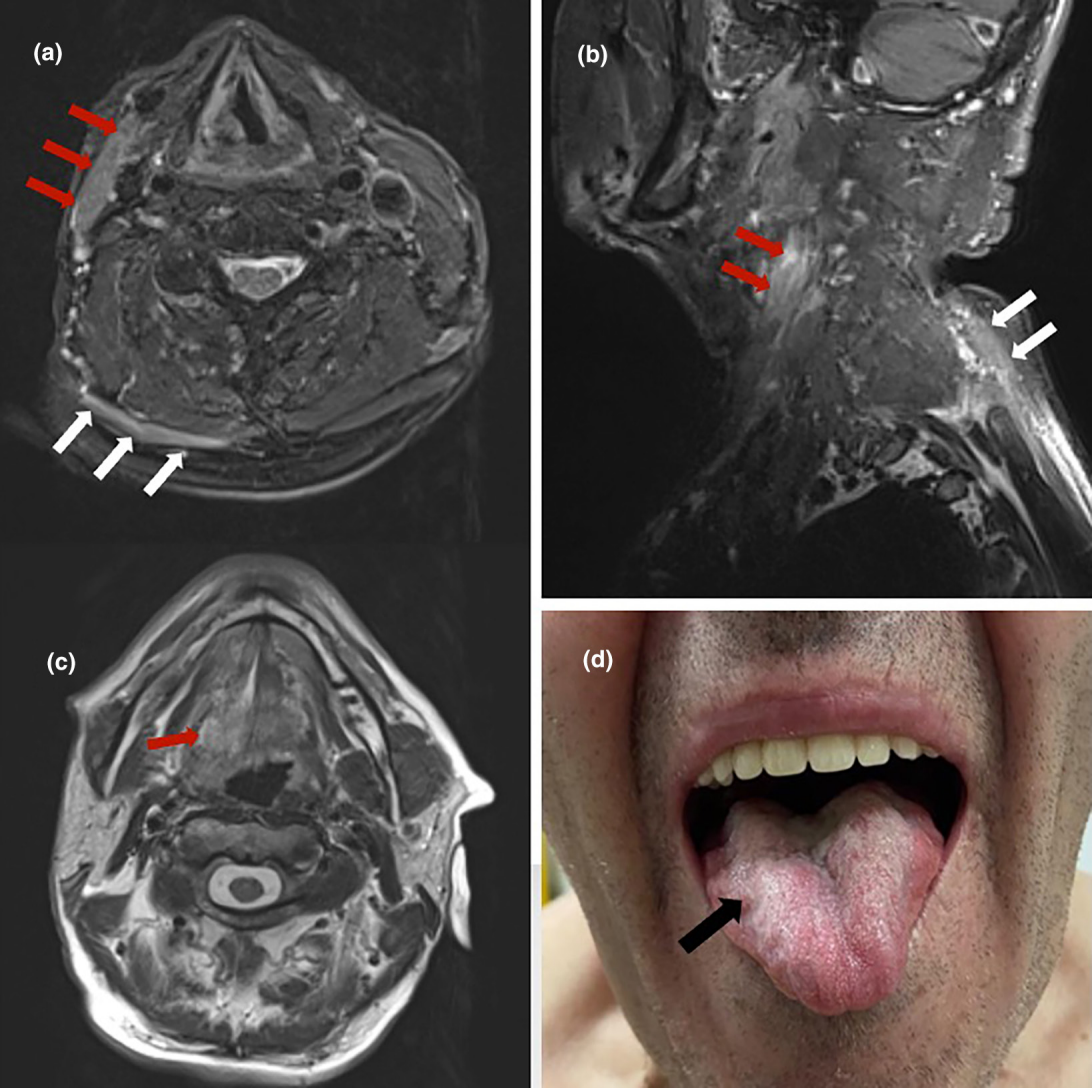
Neuroimaging findings and clinical manifestations indicating right spinal accessory nerve (XI) and hypoglossal (XII) nerve palsies. T2‐weighted and T1‐space images show right trapezius and sternocleidomastoid muscle atrophy (a, b; red arrows for sternocleidomastoid muscle and white arrows for trapezius muscle), findings compatible with spinal accessory nerve palsy. T2‐weighted magnetic resonance images (c) and neurological examination (d) revealed right‐sided atrophy of the tongue, indicating right hypoglossal nerve palsy.
